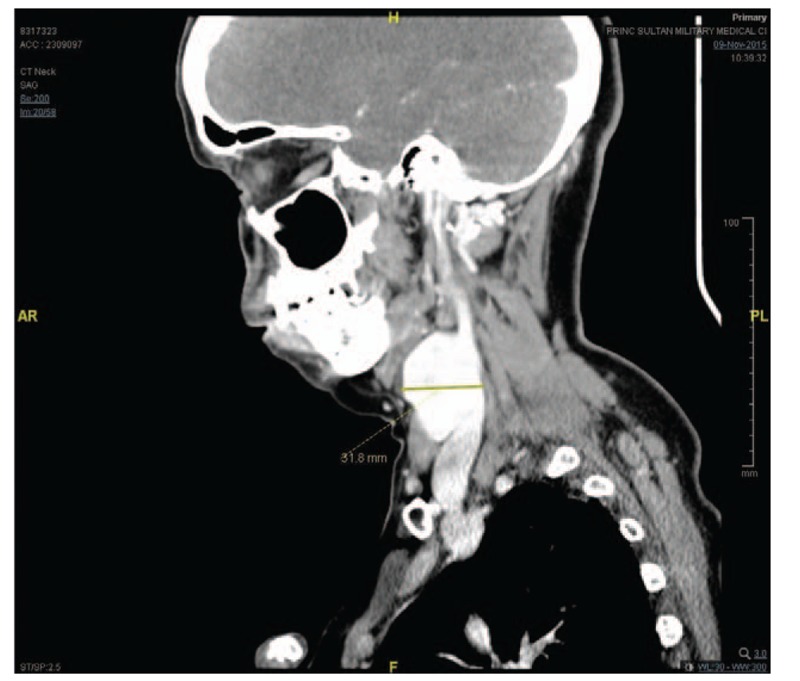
Right-sided neck saccular contrast-filled lesion visualized by contrast computed tomography.
